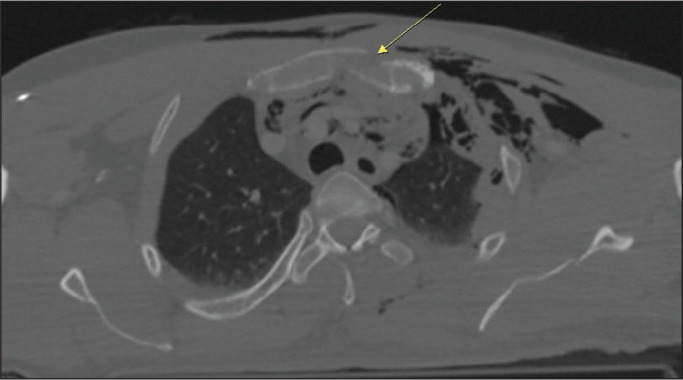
CT scan showing pneumomediastinum in a patient with a displaced fracture
